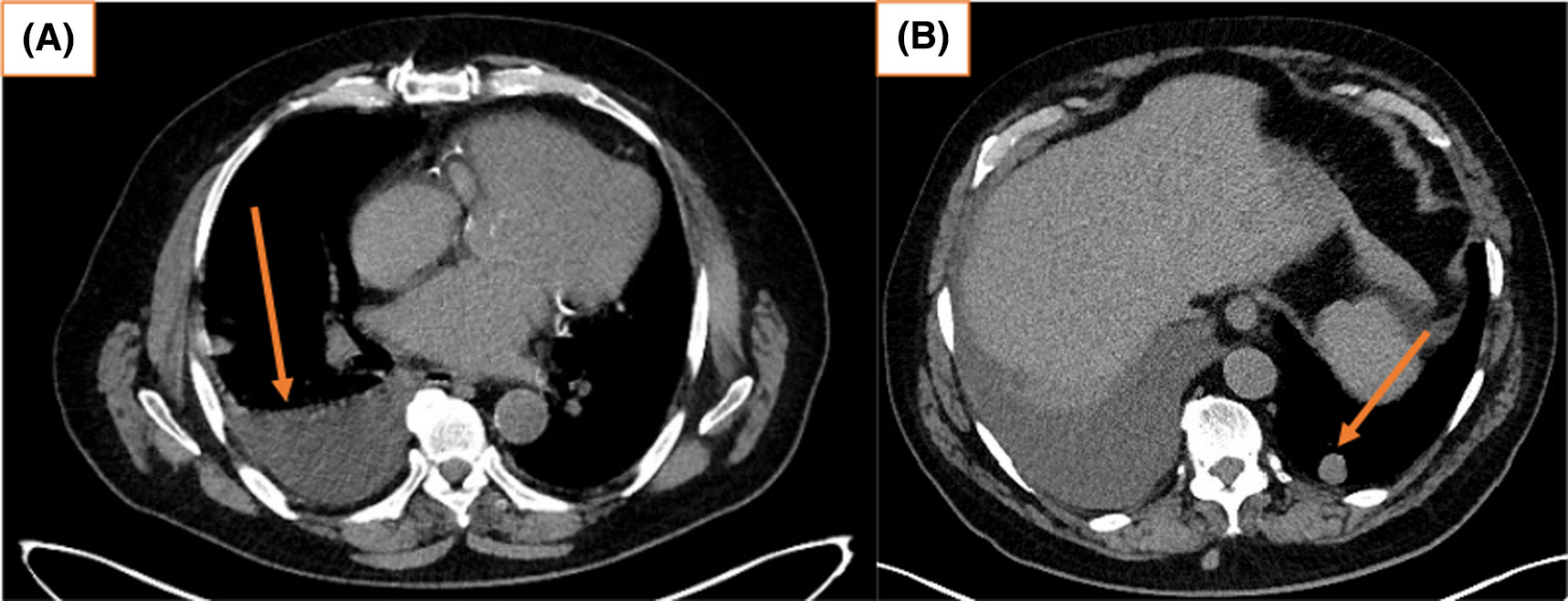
June 2022, chest CT immediately post–orchiectomy showing progression with new right pleural effusion (A) and enlarging left lung nodule (B).
